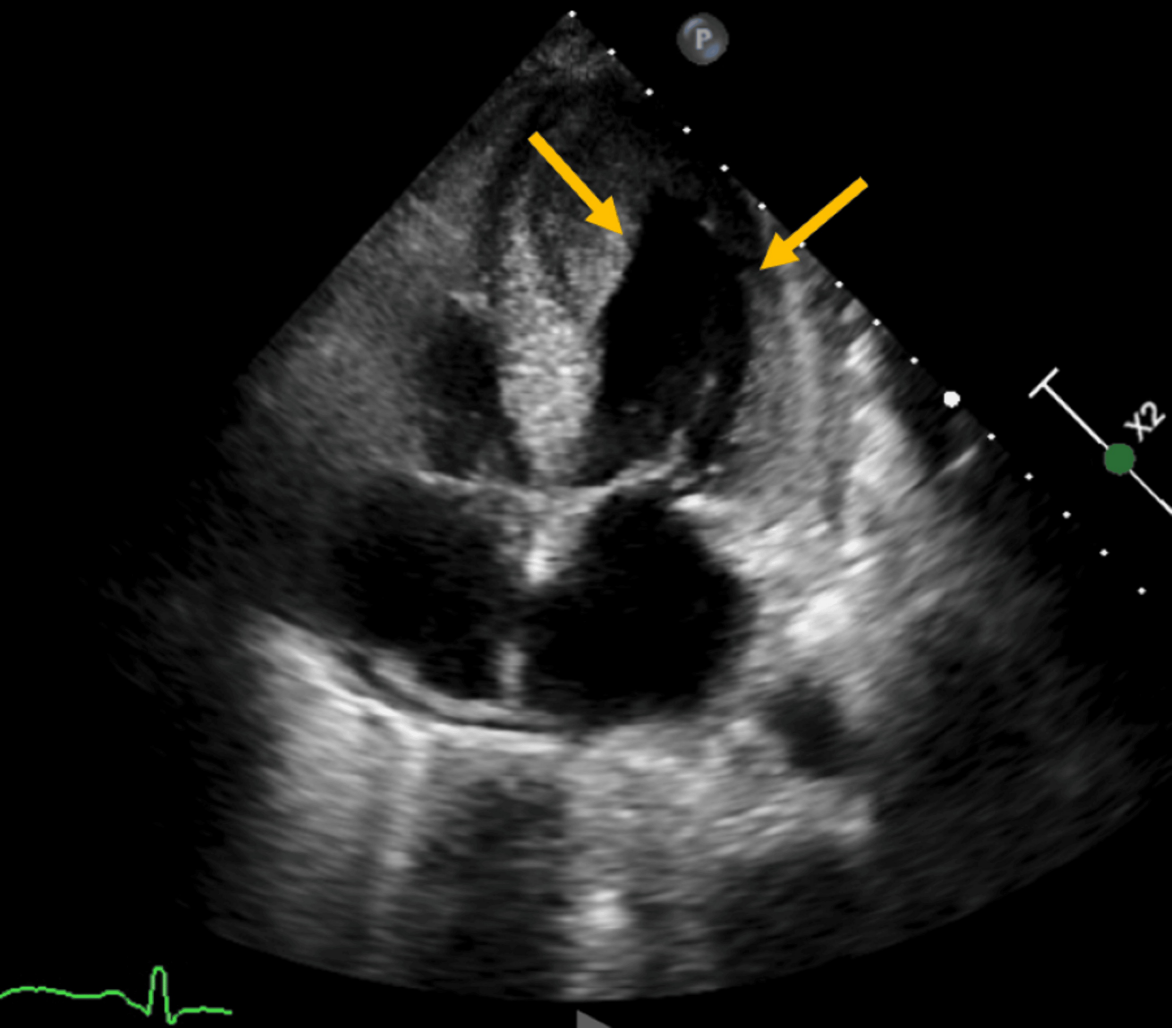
Echocardiogram on day 11 with normal ejection fraction.
